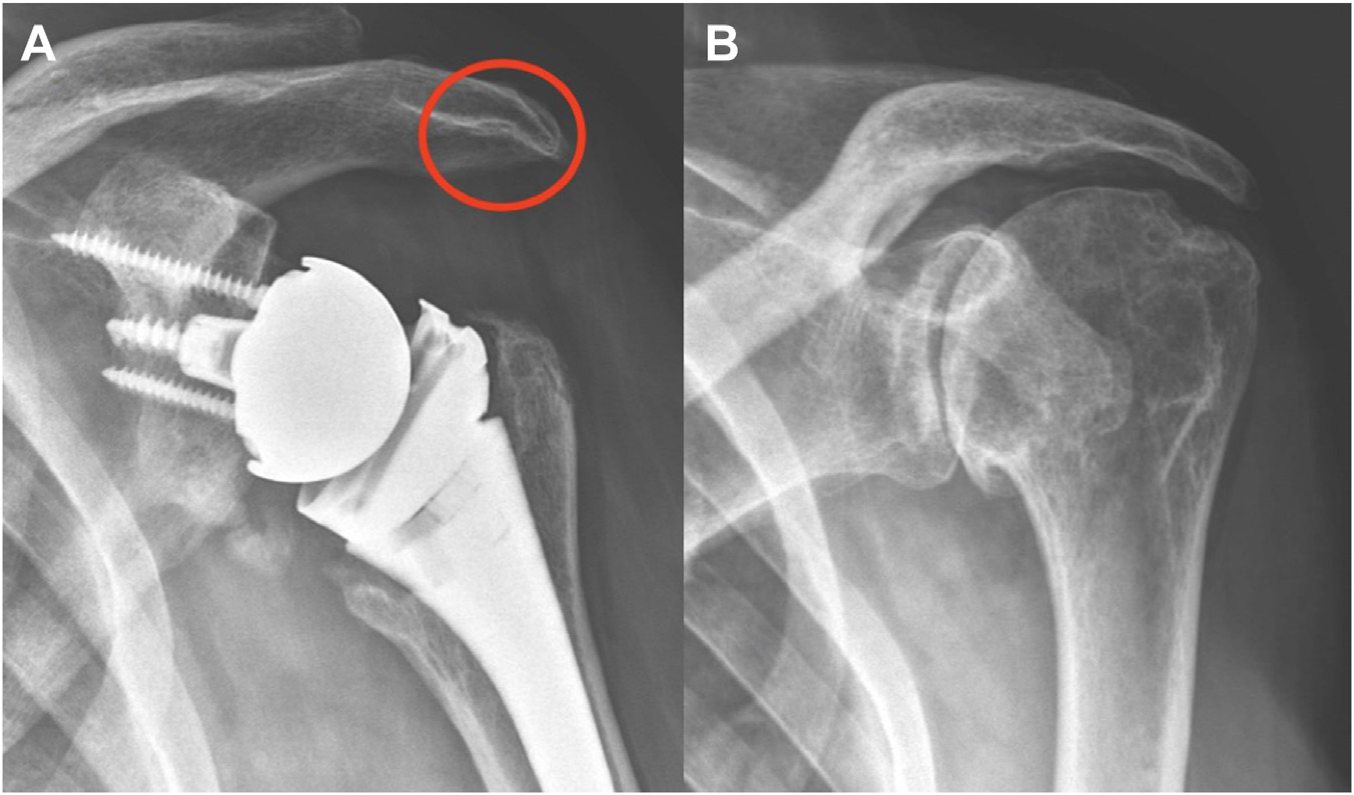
Grashey view of a left shoulder with subacromial notching as evidenced by bony erosion with sclerotic margins on the undersurface of the acromion (*circle*) at (A) final follow-up radiographs not present (B) preoperatively.
